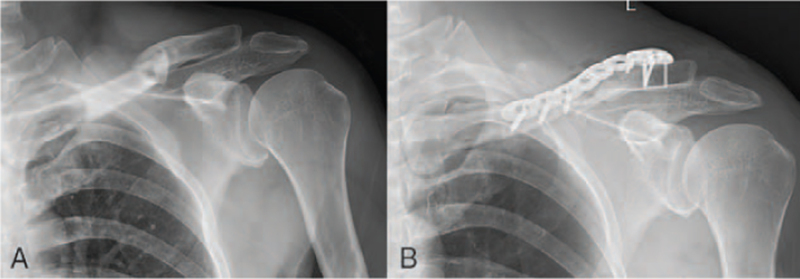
A. Preoperative anteroposterior shoulder joint DR shows a mid-third clavicle fracture without ipsilateral ACJ dislocation. B. Evidence of ACJ subluxation at the 1-month postoperative follow-up of clavicle fracture. ACJ = acromioclavicular joint; DR = digital radiography.
